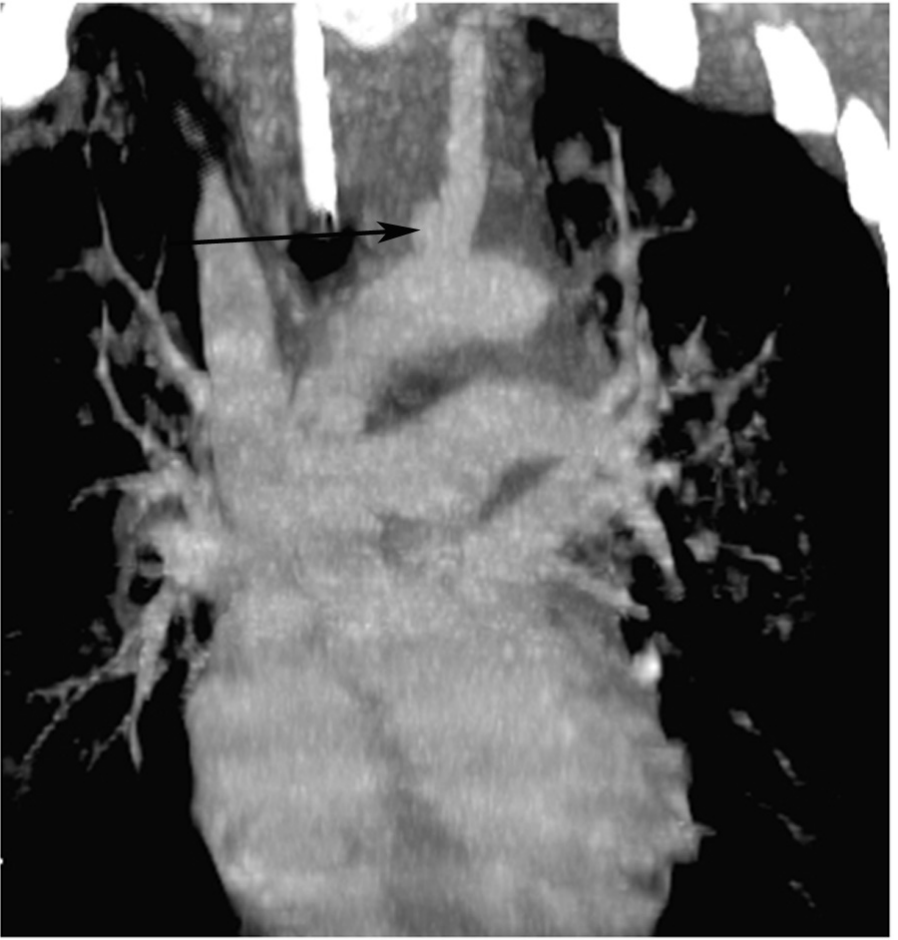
Reconstructed computed tomography with contrast demonstrating a pseudoaneurysm at the origin of the left common carotid artery (arrow).
